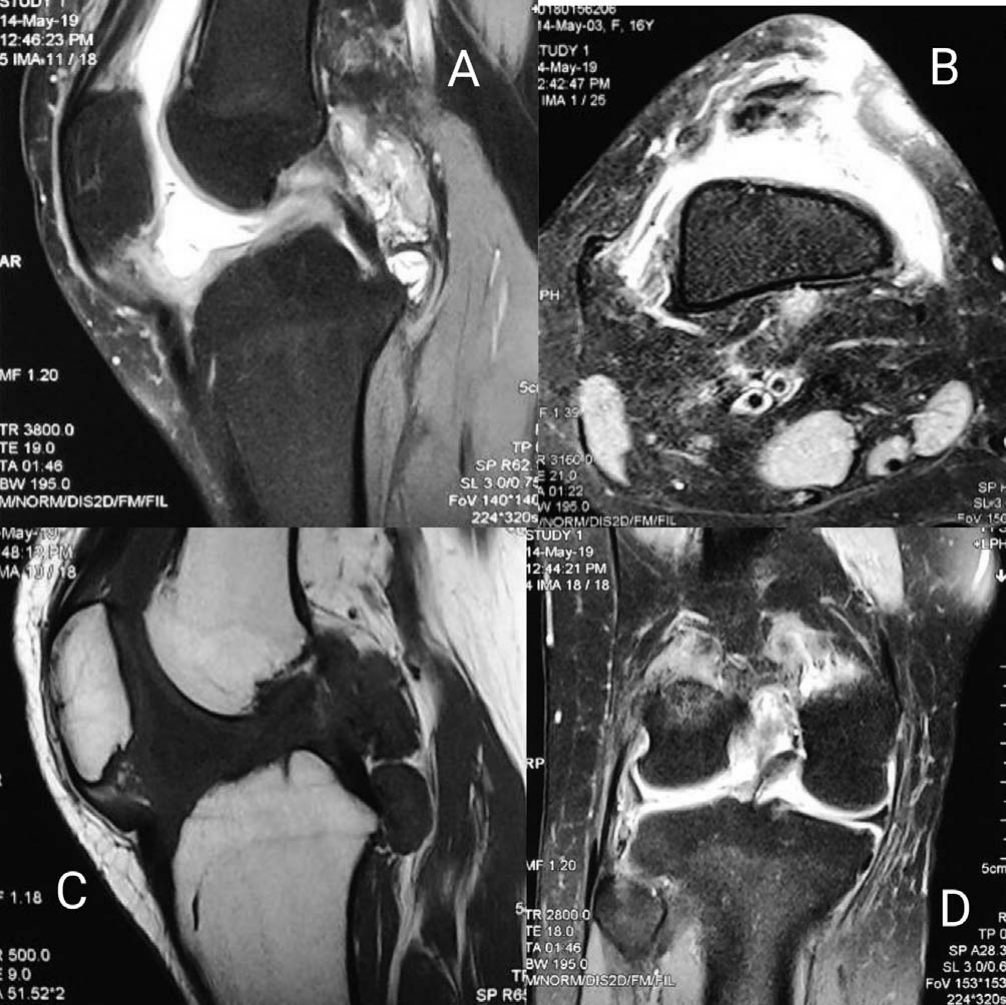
Figure showing MRI sections of a patient with pigmented villo-nodular synovitis of knee (A – sagittal STIR, B – axial STIR, C – sagittal T1, D – coronal STIR images showing nodular synovial thickening with foci of blooming within patellar tendon, Hoffa’s pad of fat, quadriceps tendon, and popliteal fossa).
